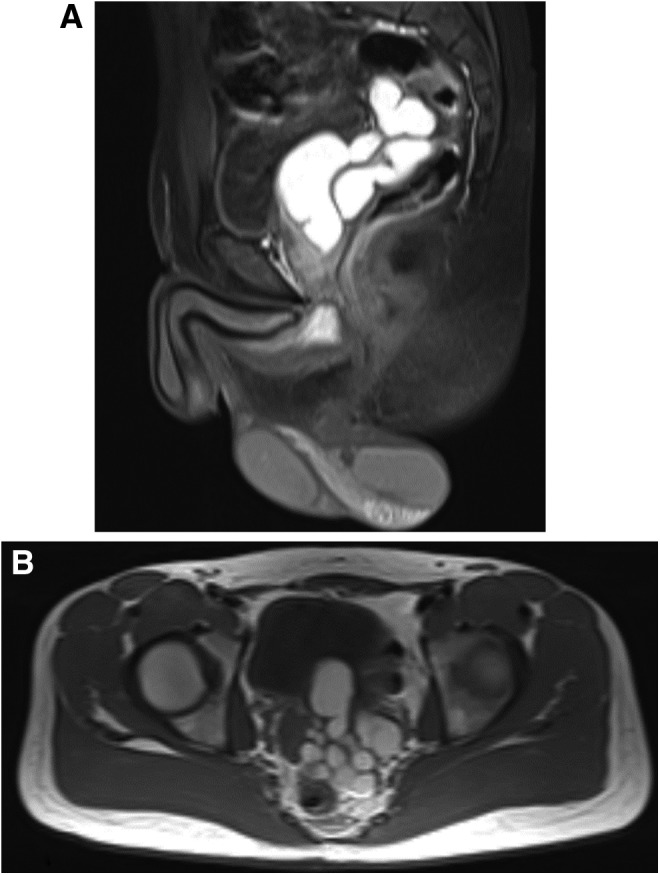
Sagittal (A) and axial (B) MRI images show a large (∼5 × 6.5 × 8 cm) tubular fluid-filled structure originating from the left seminal vesicle consistent with seminal vesicle cyst. A small left ureteral stump is evident, consistent with rudimentary ureter.
